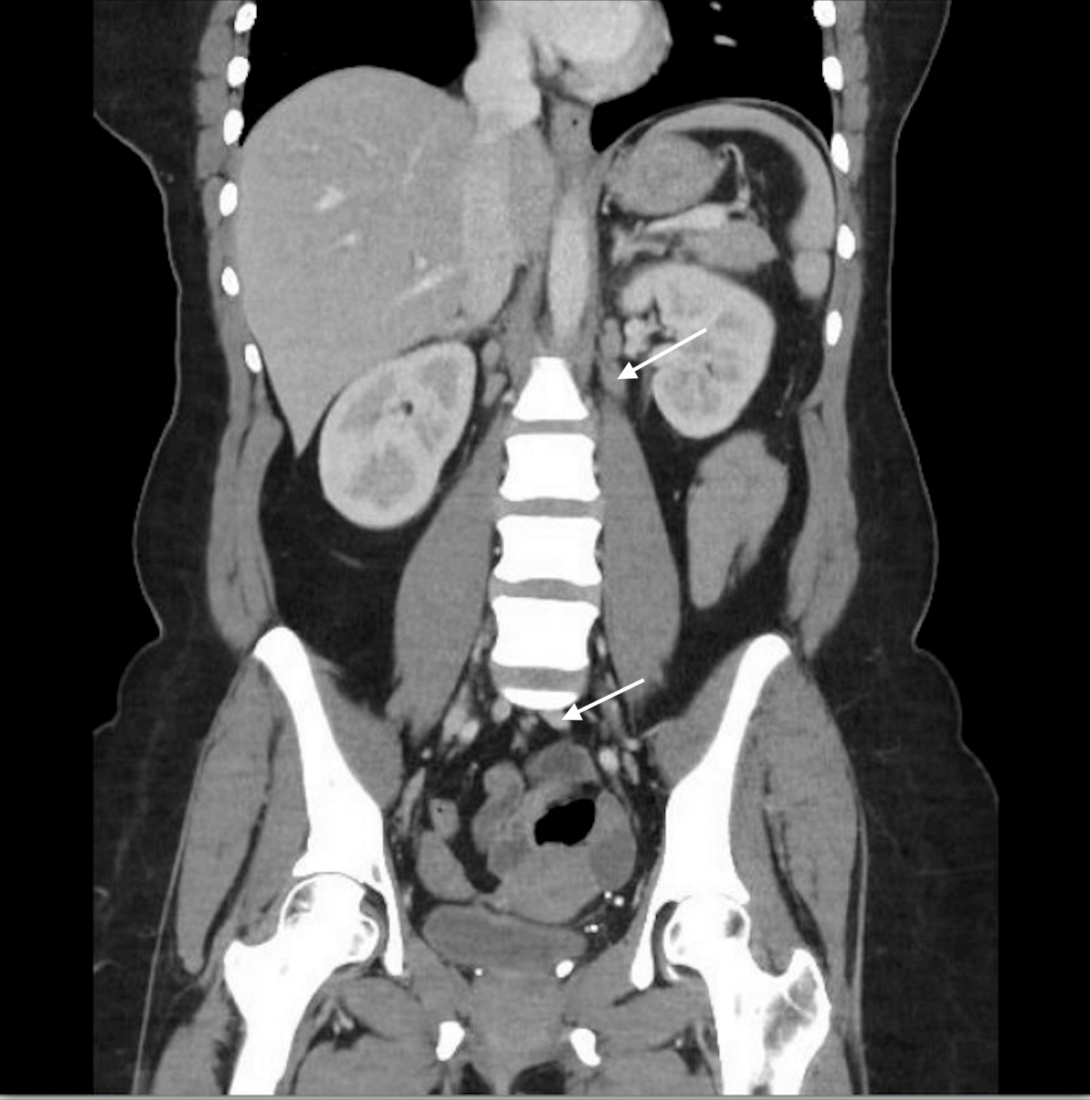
Coronal computed tomography image of the abdomen CT = computed tomography. Coronal CT image of the abdomen demonstrating enlarged retroperitoneal (upper arrow) and mesenteric (lower arrow) lymph nodes, consistent with disseminated histoplasmosis.
